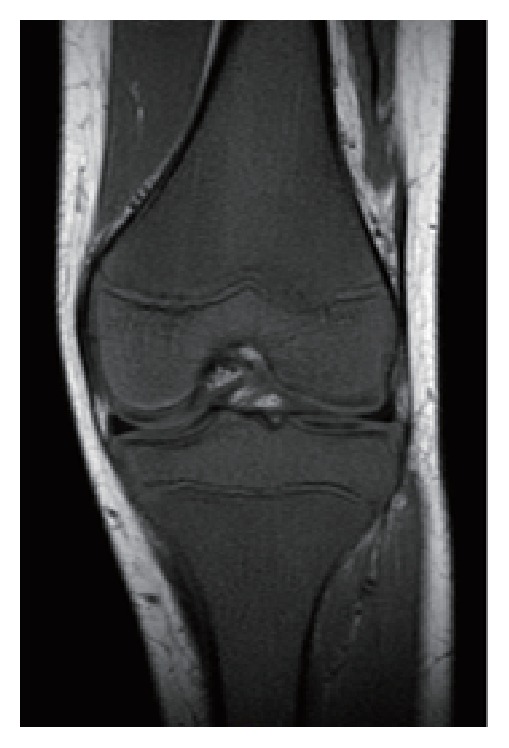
MRI findings in case 2. At 4 months after the disease onset, T1-weighted image depicts low signal intensity in the right femoral and tibial bone marrow.
